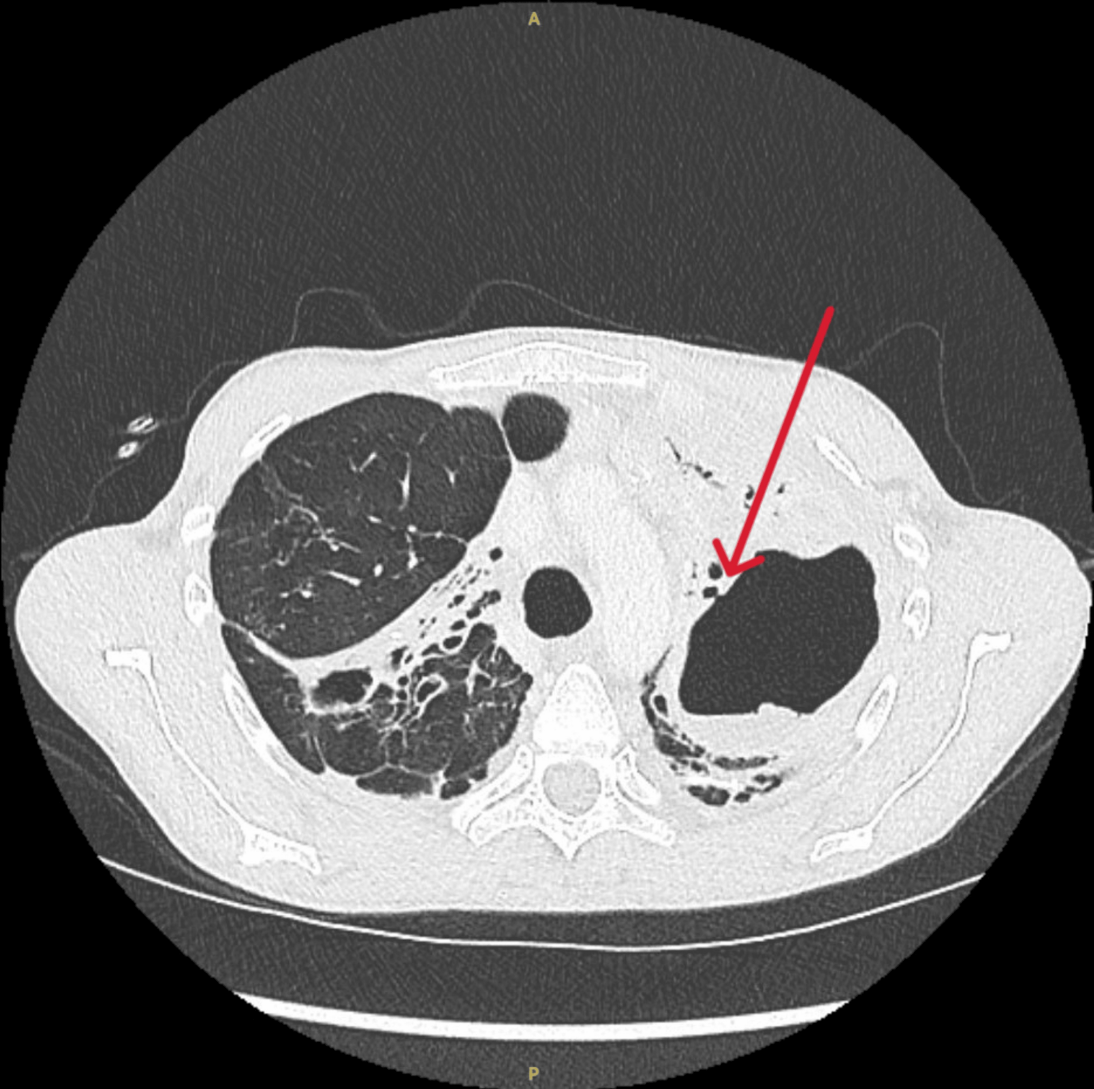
Computed tomography of the chest with one of the three fistulae.
